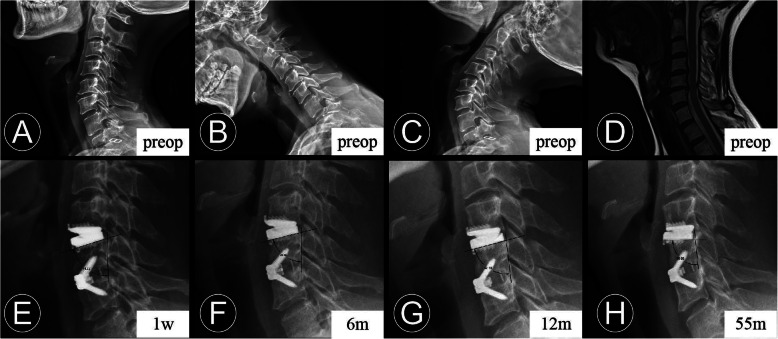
A 51-year-old woman who underwent contiguous 2-level hybrid surgery at C4-C6. b and c Preoperative dynamic radiographs show that a loss of intervertebral disc height and decrease of segmental mobility occurred at C5–6. Thus, fusion was performed at C5–6 and cervical disc arthroplasty was performed at C4–5. e and f The immediate and 6-month postoperative lateral radiographs show the peri-prosthesis bone loss was found at the replacement level. e, f, g, and h The measurement method for the degree between the prosthesis position and posterior vertebral line. The postoperative lateral radiographs obtained at 1 week, 6 months, 12 months, and 55 months after surgery show the progression of implant subsidence
